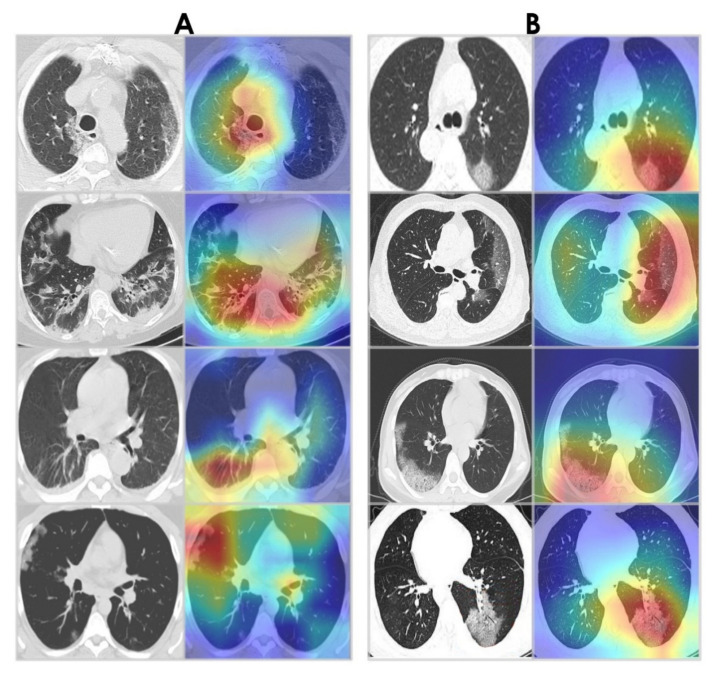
Grad-CAM visualizations. (A) Sample CT images from the SARS-CoV-2 CT-scan dataset [42]. (B) Sample CT images from the COVID-CT dataset [22].
